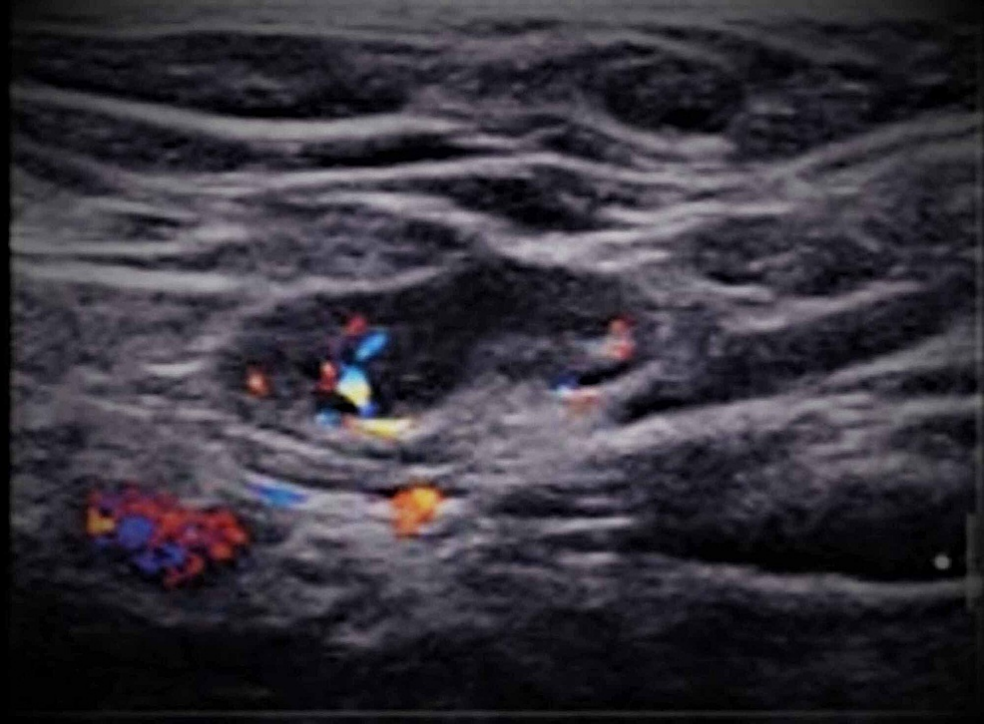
Ultrasound of the left axilla showing enlarged node with normal architecture and blood flow.
